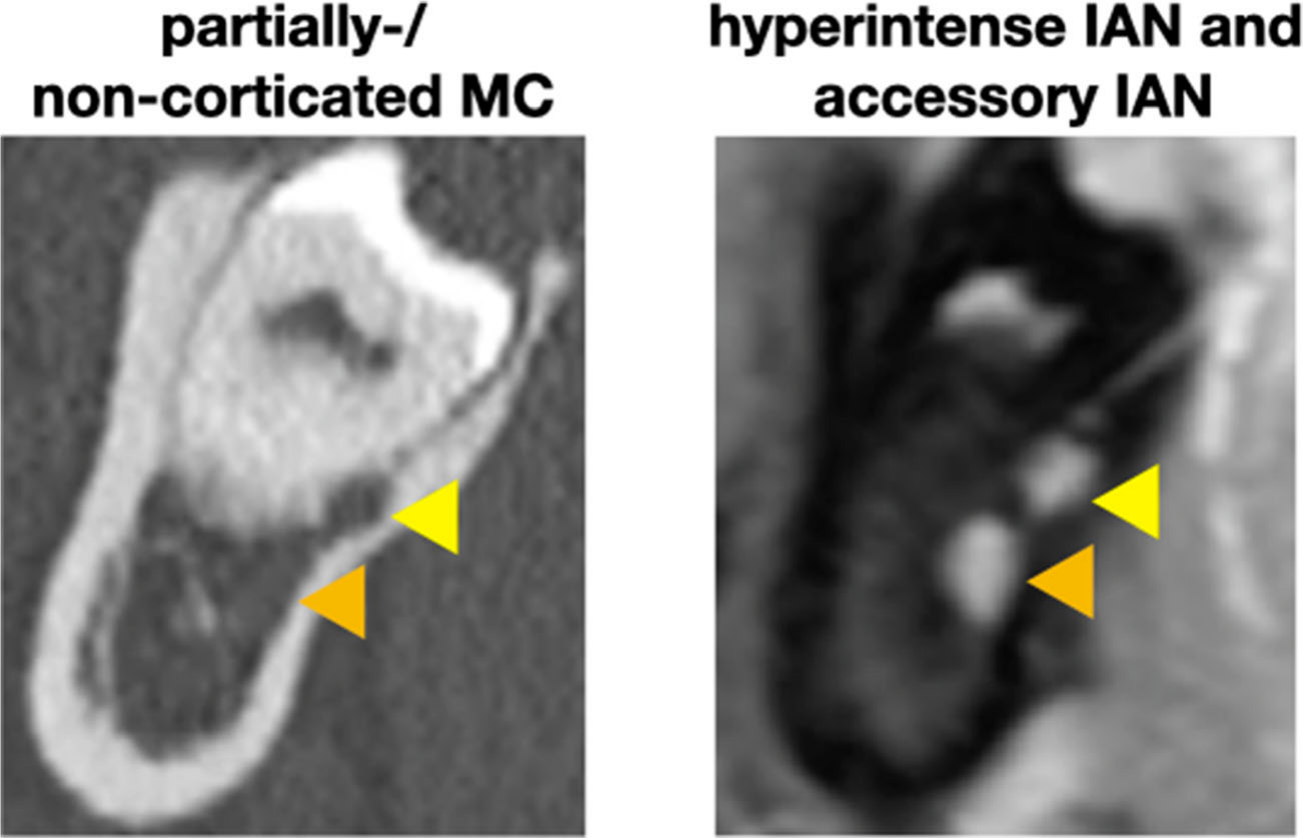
A case with the MC lacking a bony wall and with an accessory IAN. Left (CT): the MC (indicated by the orange arrow) is hardly visible and the accessory IAN (indicated by the yellow arrow) could be missed; right (MRI): both NVB appear clearly hyperintense
